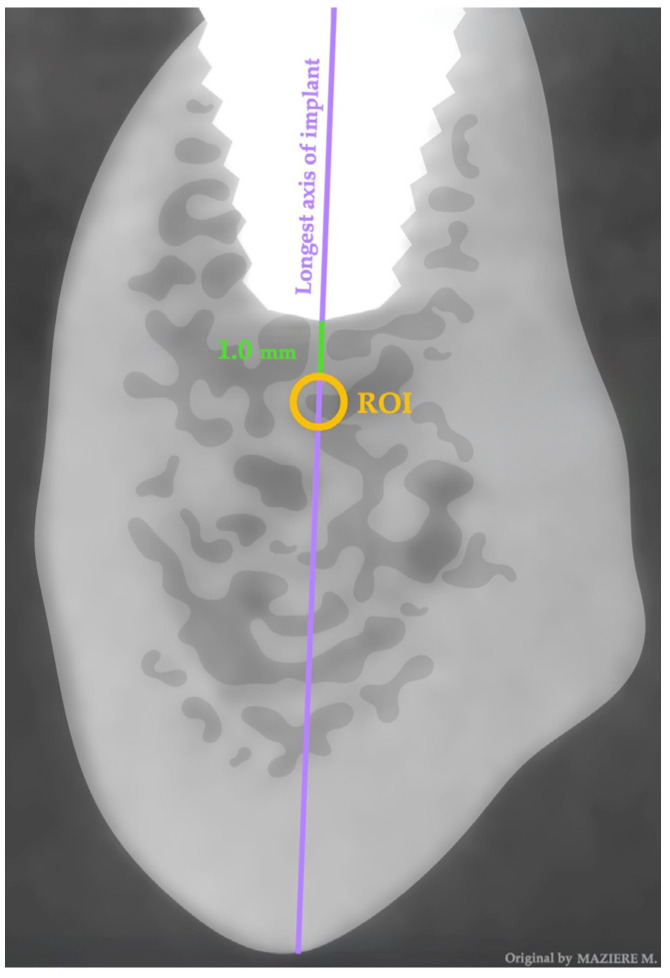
CBCT section showing radiographic evaluation methodology.
